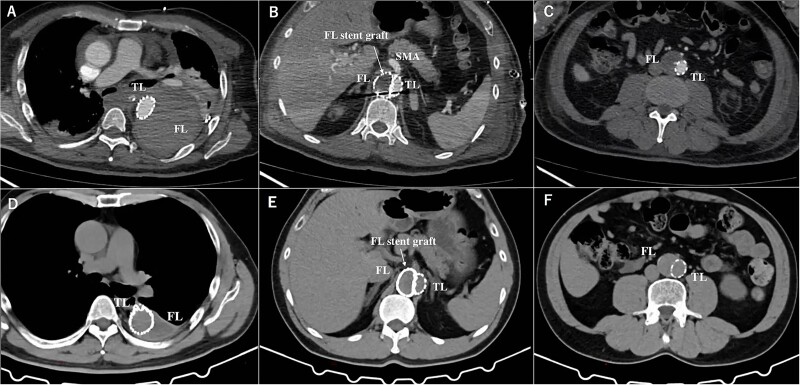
The postoperative contrasted computed tomography at thoracic level (A), at SMA level (B), at abdominal level (C). The computed tomography three years after the operation at thoracic level (D), at SMA level (E), at abdominal level (F). TL: true lumen FL: false lumen RA: renal artery CA: celiac artery SMA: superior mesenteric artery CIA: common iliac artery.
